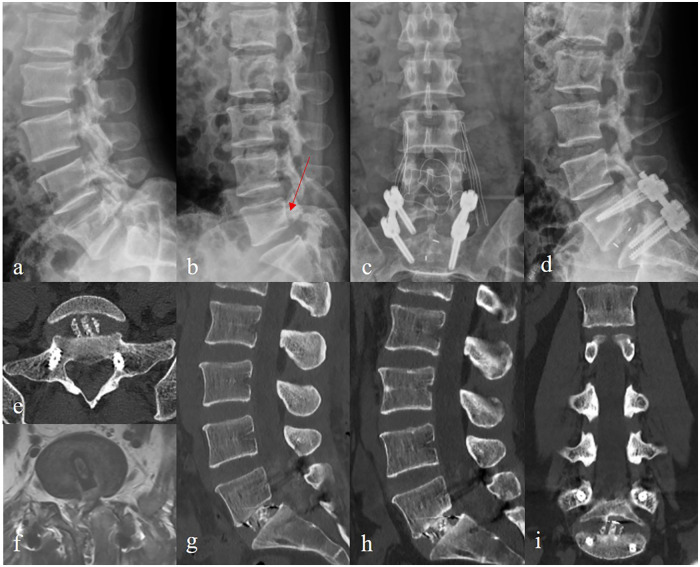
(a,b) A 50-year-old male patient showed L5 isthmic spondylolisthesis with dynamic instability on flexion-extension x-rays (translation >3 mm and angulation >10°). (c–f) Postoperative imaging (x-ray, CT, and MRI axial views) at 2 days post-UBE-LIF, demonstrating satisfactory internal fixation and one cage in position. (g) CT at 6 months post-surgery showing no solid fusion at L5-S1. (h,i) CT at 1 year post-surgery confirming solid fusion at L5-S1.
